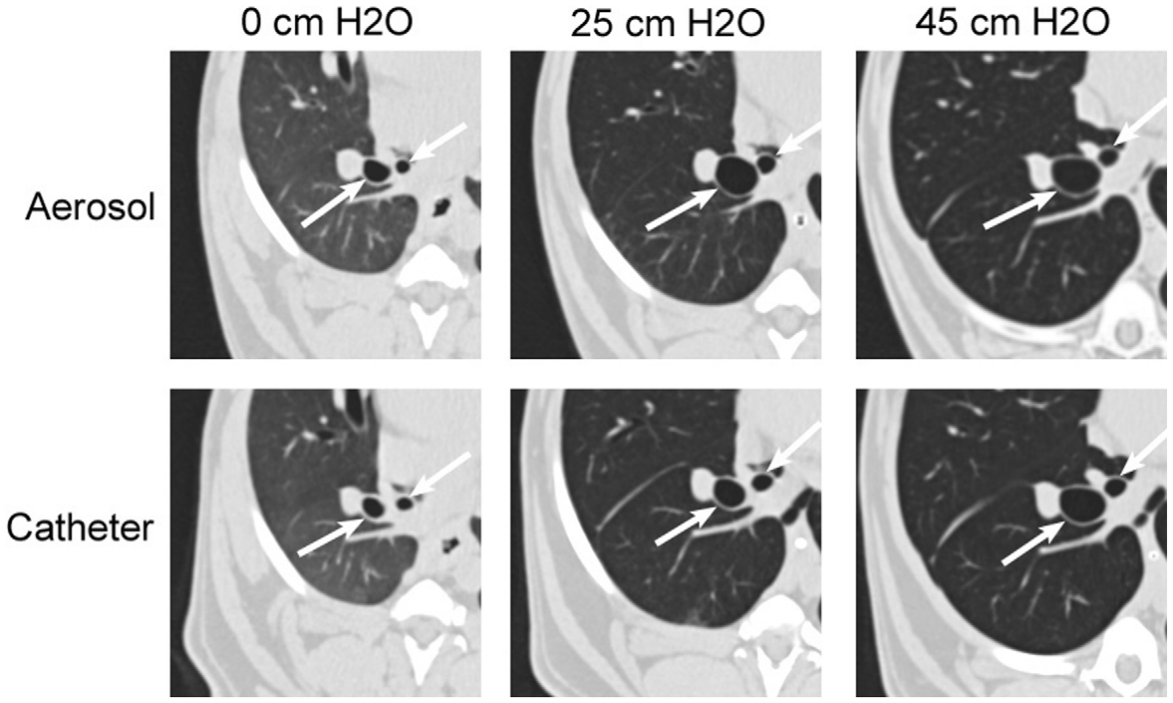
Representative HRCT images at three pressures with either the local catheter or whole lung aerosol challenge. Two individual airways identified by the arrows are seen in all the images. The airway sizes at 0 and 45 cm H_2_O appear similar with either challenge, but at 25 cm H_2_O, the airways with the catheter stimulation are clearly smaller than with the aerosol challenge.
